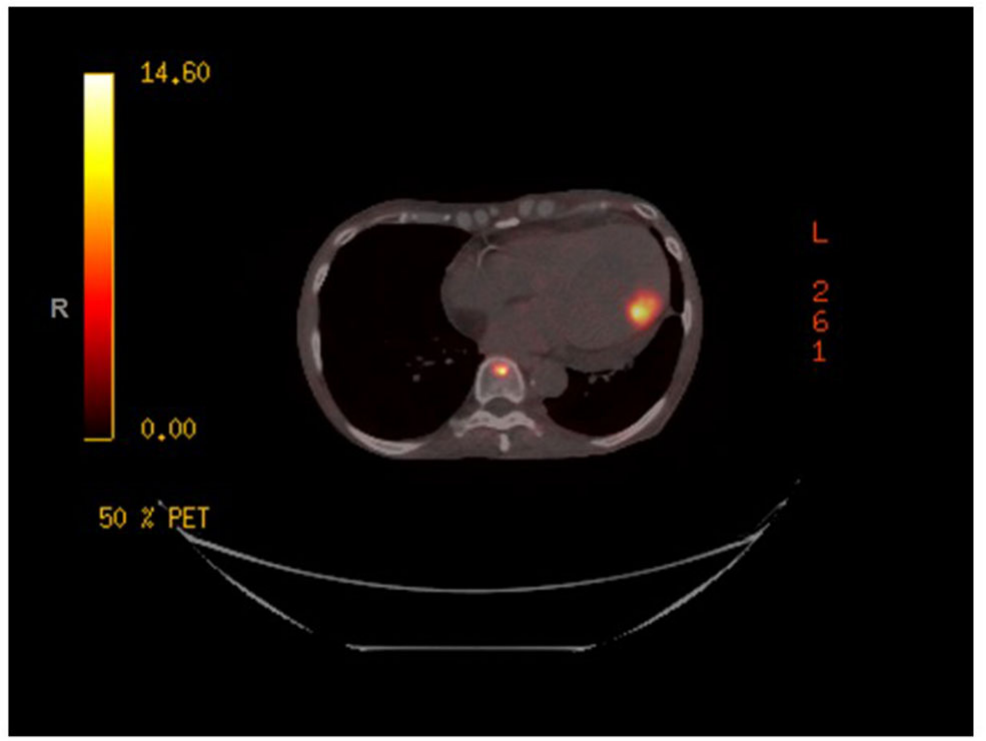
Gallium-68 dotatate PET/CT scan demonstrating avidity in the left ventricular myocardium.
